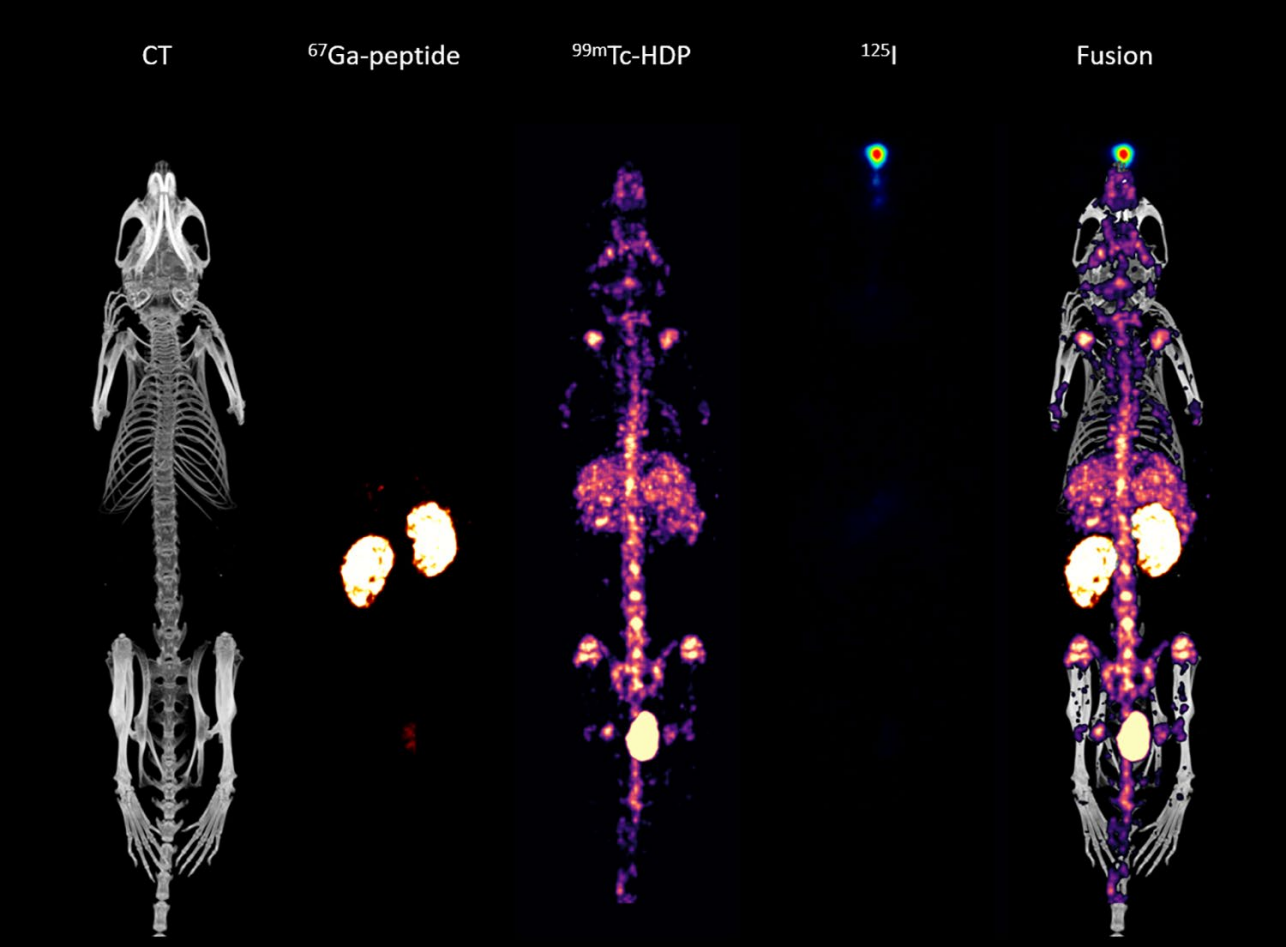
Simultaneous SPECT/CT images obtained for three different tracers labeled with ^99m^Tc, ^67^Ga and ^125^I. Separate reconstruction has been obtained for each isotope.
